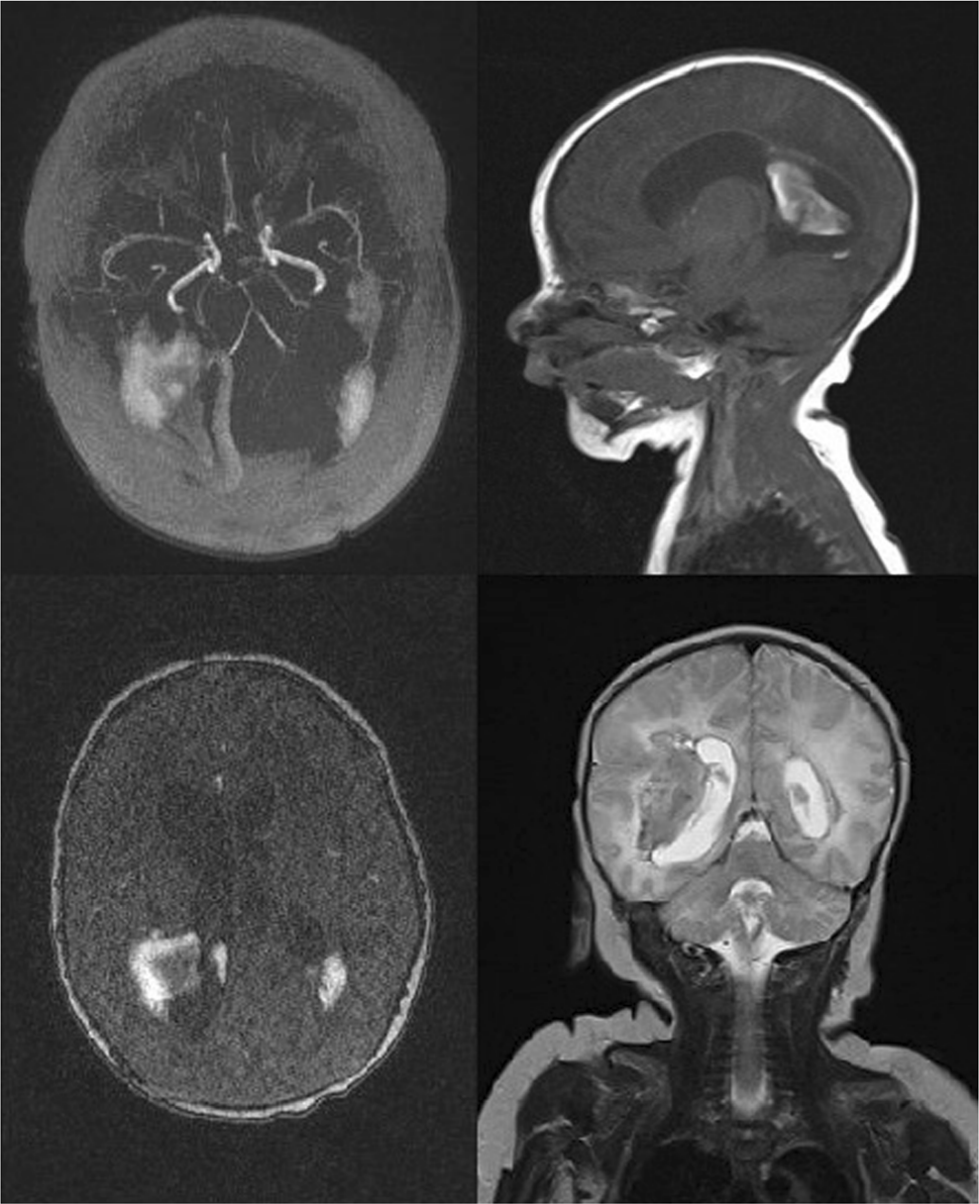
Brain magnetic resonance imaging of the neonate reported as case 1. The right hemisphere of the brain on the border of the temporoparietal lobes, an intracerebral hematoma, second in the left temporal lobe
